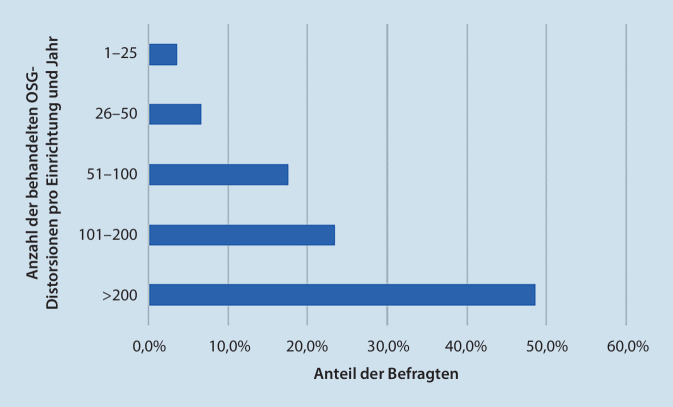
In der klinischen Untersuchung im Rahmen der Erstvorstellung werden der Drawer-Test (Talusvorschub/Schubladentest) (89,5 %) und der Inversions‑/Eversions-Stress-Test (81,6 %) am häufigsten durchgeführt. Für die Entscheidungsfindung zur Röntgendiagnostik fanden 43,3 % die Ottawa Ankle Rules hilfreich, 27,3 % manchmal hilfreich und 29,4 % wenig oder nicht hilfreich. 88,1 % gaben an, *dass sie im Rahmen der Erstvorstellung regelhaft ein Röntgenbild ohne Belastung anfertigen würden, ohne dass dies im Rahmen des Fragebogens weiter präzisiert wurde.* Zweithäufigste Diagnostik ist die Sonographie (26,5 %), gefolgt von Röntgenbildern im Stand (10,4 %). Bei *klinischem *Verdacht auf höhergradige Verletzungen führen 90 % der Befragten eine Magnetresonanztomographie(MRT)-Untersuchung durch, 37,2 % eine Computertomographie (CT). Dies spiegelt sich auch darin wider, dass 91,6 % die MRT für sehr hilfreich oder hilfreich bei Verdacht auf höhergradige Verletzungen einschätzen. Die CT wird von 51,8 % für mindestens hilfreich gehalten. Die digitale Volumentomographie (DVT) wird nur von 16,9 % als hilfreich oder sehr hilfreich eingeschätzt, gehaltene Aufnahmen von 14,3 %.
